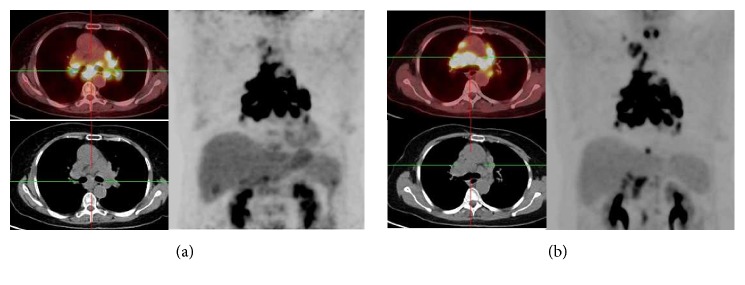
Results for patient 17, a 46-y-old woman with symptomatic sarcoidosis. (a) A baseline 18F-FDG PET image revealed increased activity in mediastinal lymph nodes. (b) On the follow-up examination performed at 3 months, uptake in the mediastinal lymph nodes increased.
